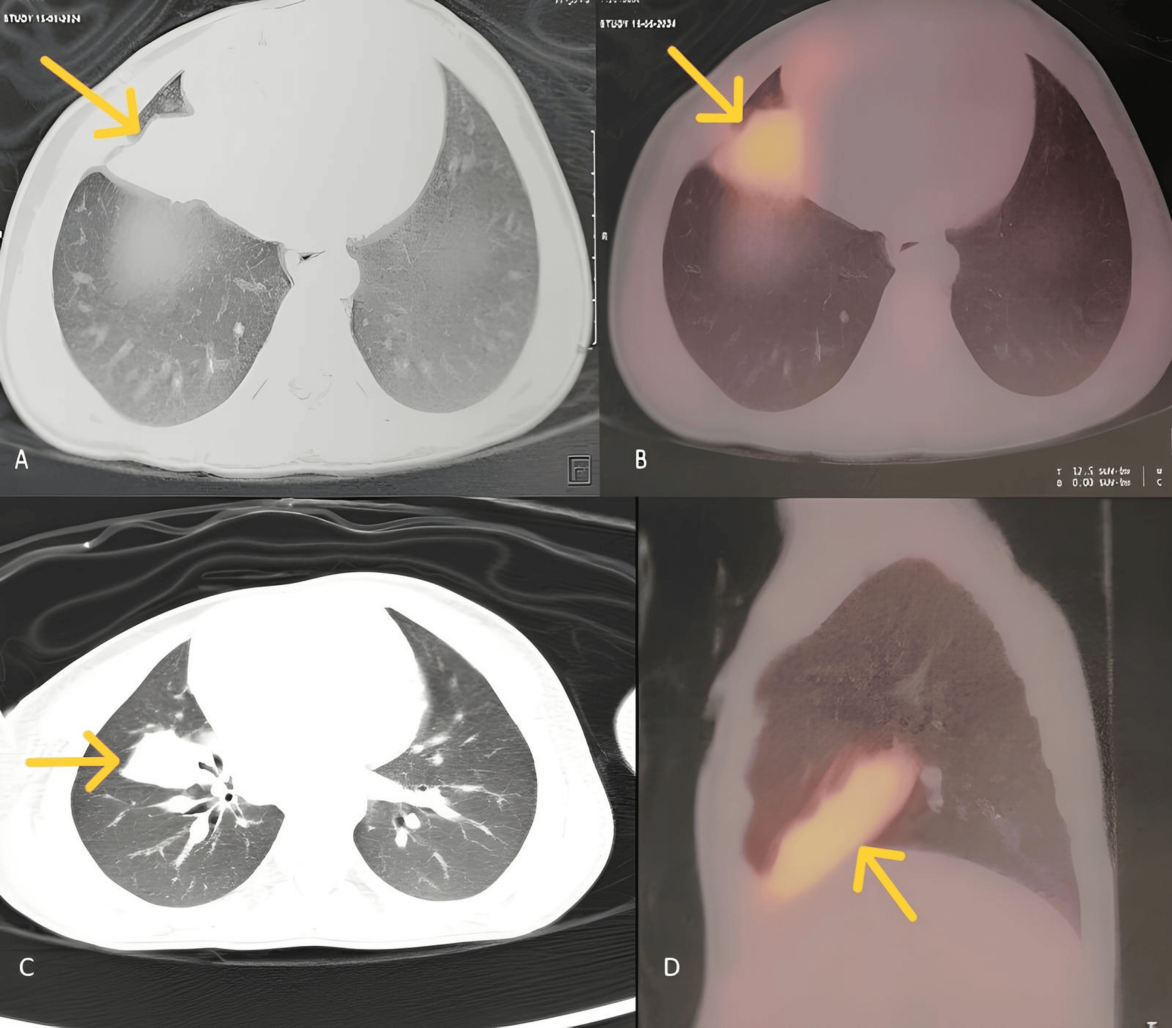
Imaging results (A) Axial view of contrast-enhanced CT of thorax showing an enhancing interfissural mass (yellow arrow) in the middle lobe of the right lung (size 1.3 x 2.1 x 3.7 cm). (B) A FDG-avid lesion with a maximum standard uptake value (SUV max) of 8.0 (yellow arrow). (C) Interfissural consolidation with another possibility of mass, in the right middle lobe (yellow arrow). (D) Saggital section view showing oblong mass-like lesion in right middle lobe (yellow arrow), with FDG uptake CT: computed tomography; FDG: fluoro-deoxy-glucose
